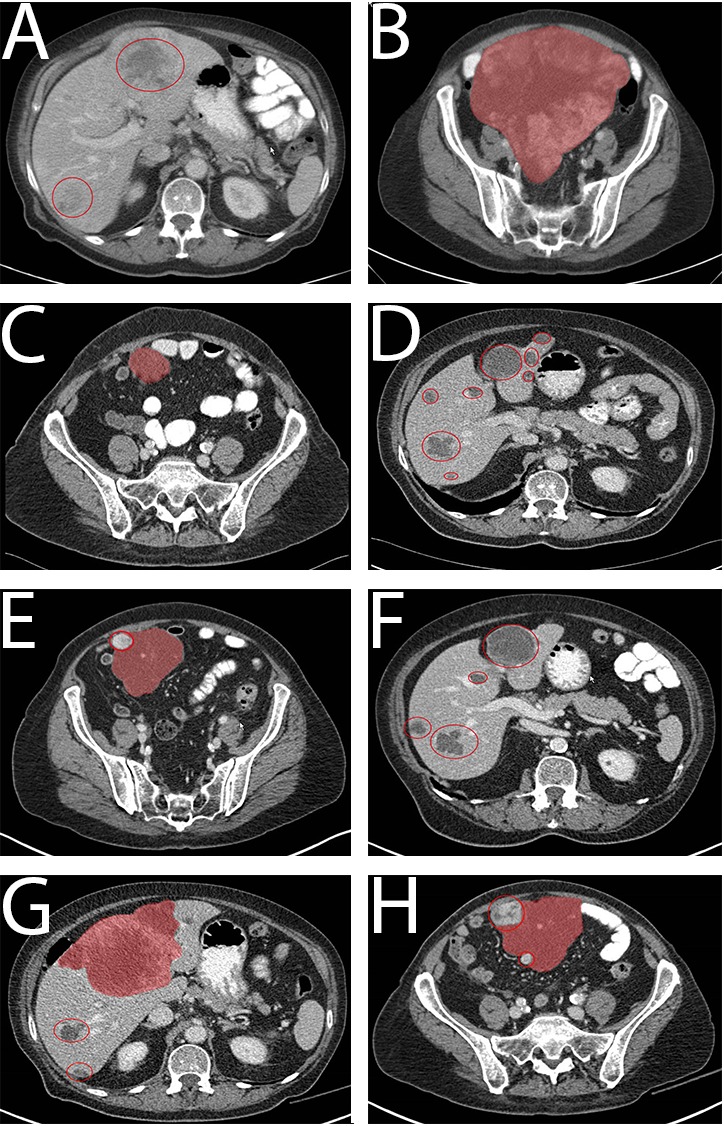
CT images of patient 2, left column images of pelvis, right column images of liver at the same time Primary tumor (red area), metastases (red circles). A. Pretreatment, large tumor in the lower abdomen. B. Pretreatment, multiple liver metastases. C. 24 months imatinib, treatment response. D. 24 months imatinib, demarquation of metastases. E. 30 months imatinib, progressive tumor nodule (red circle). F. 30 months imatinib, progression liver metastases. G. 33 months, multiple progressive nodules (red circle). H. 33 months, progression of liver metastases.
